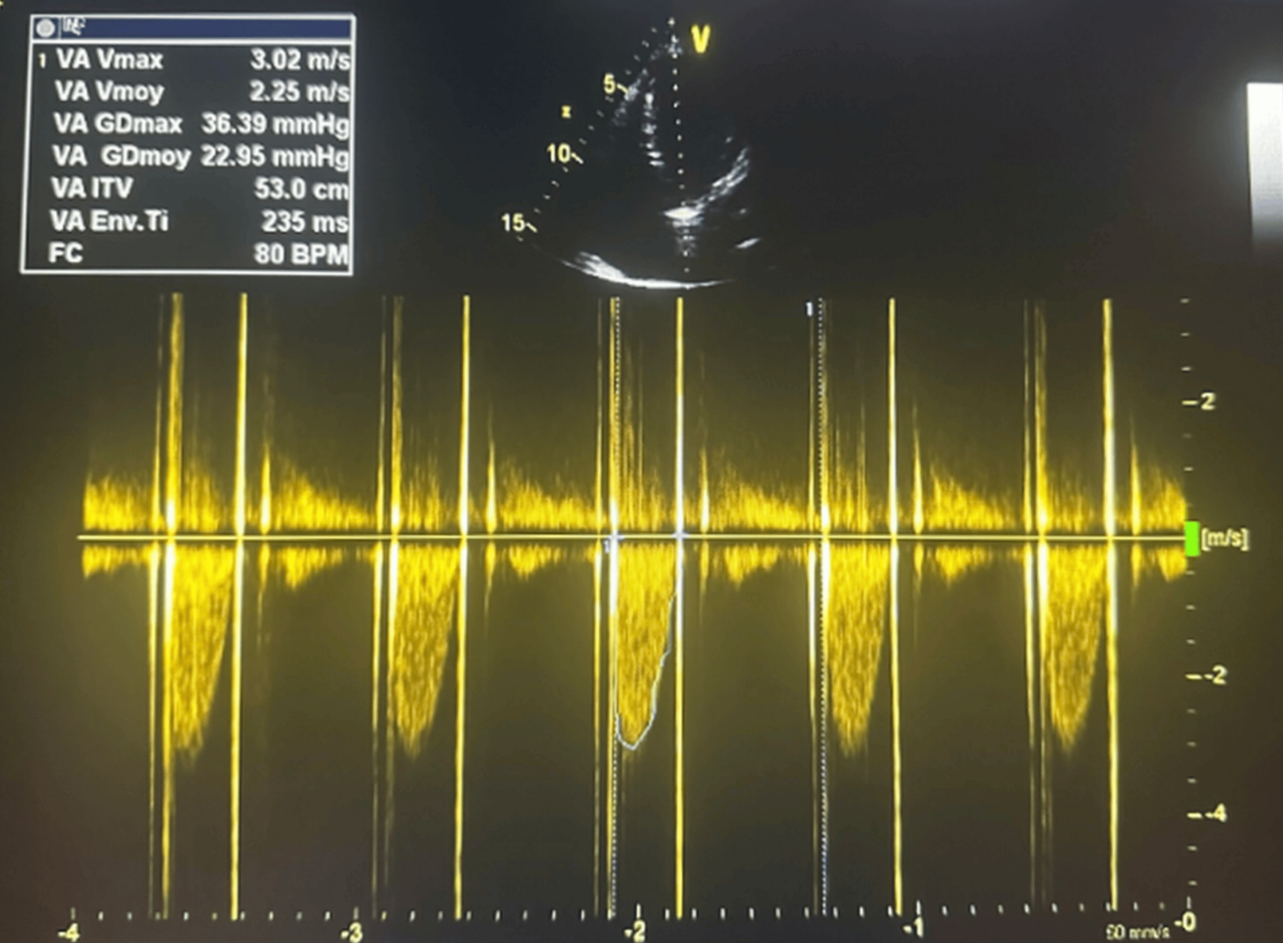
Doppler echocardiography after thrombolysis demonstrating normalization of transaortic gradients; Restoration of normal flow confirms successful thrombolytic resolution of the obstruction.
